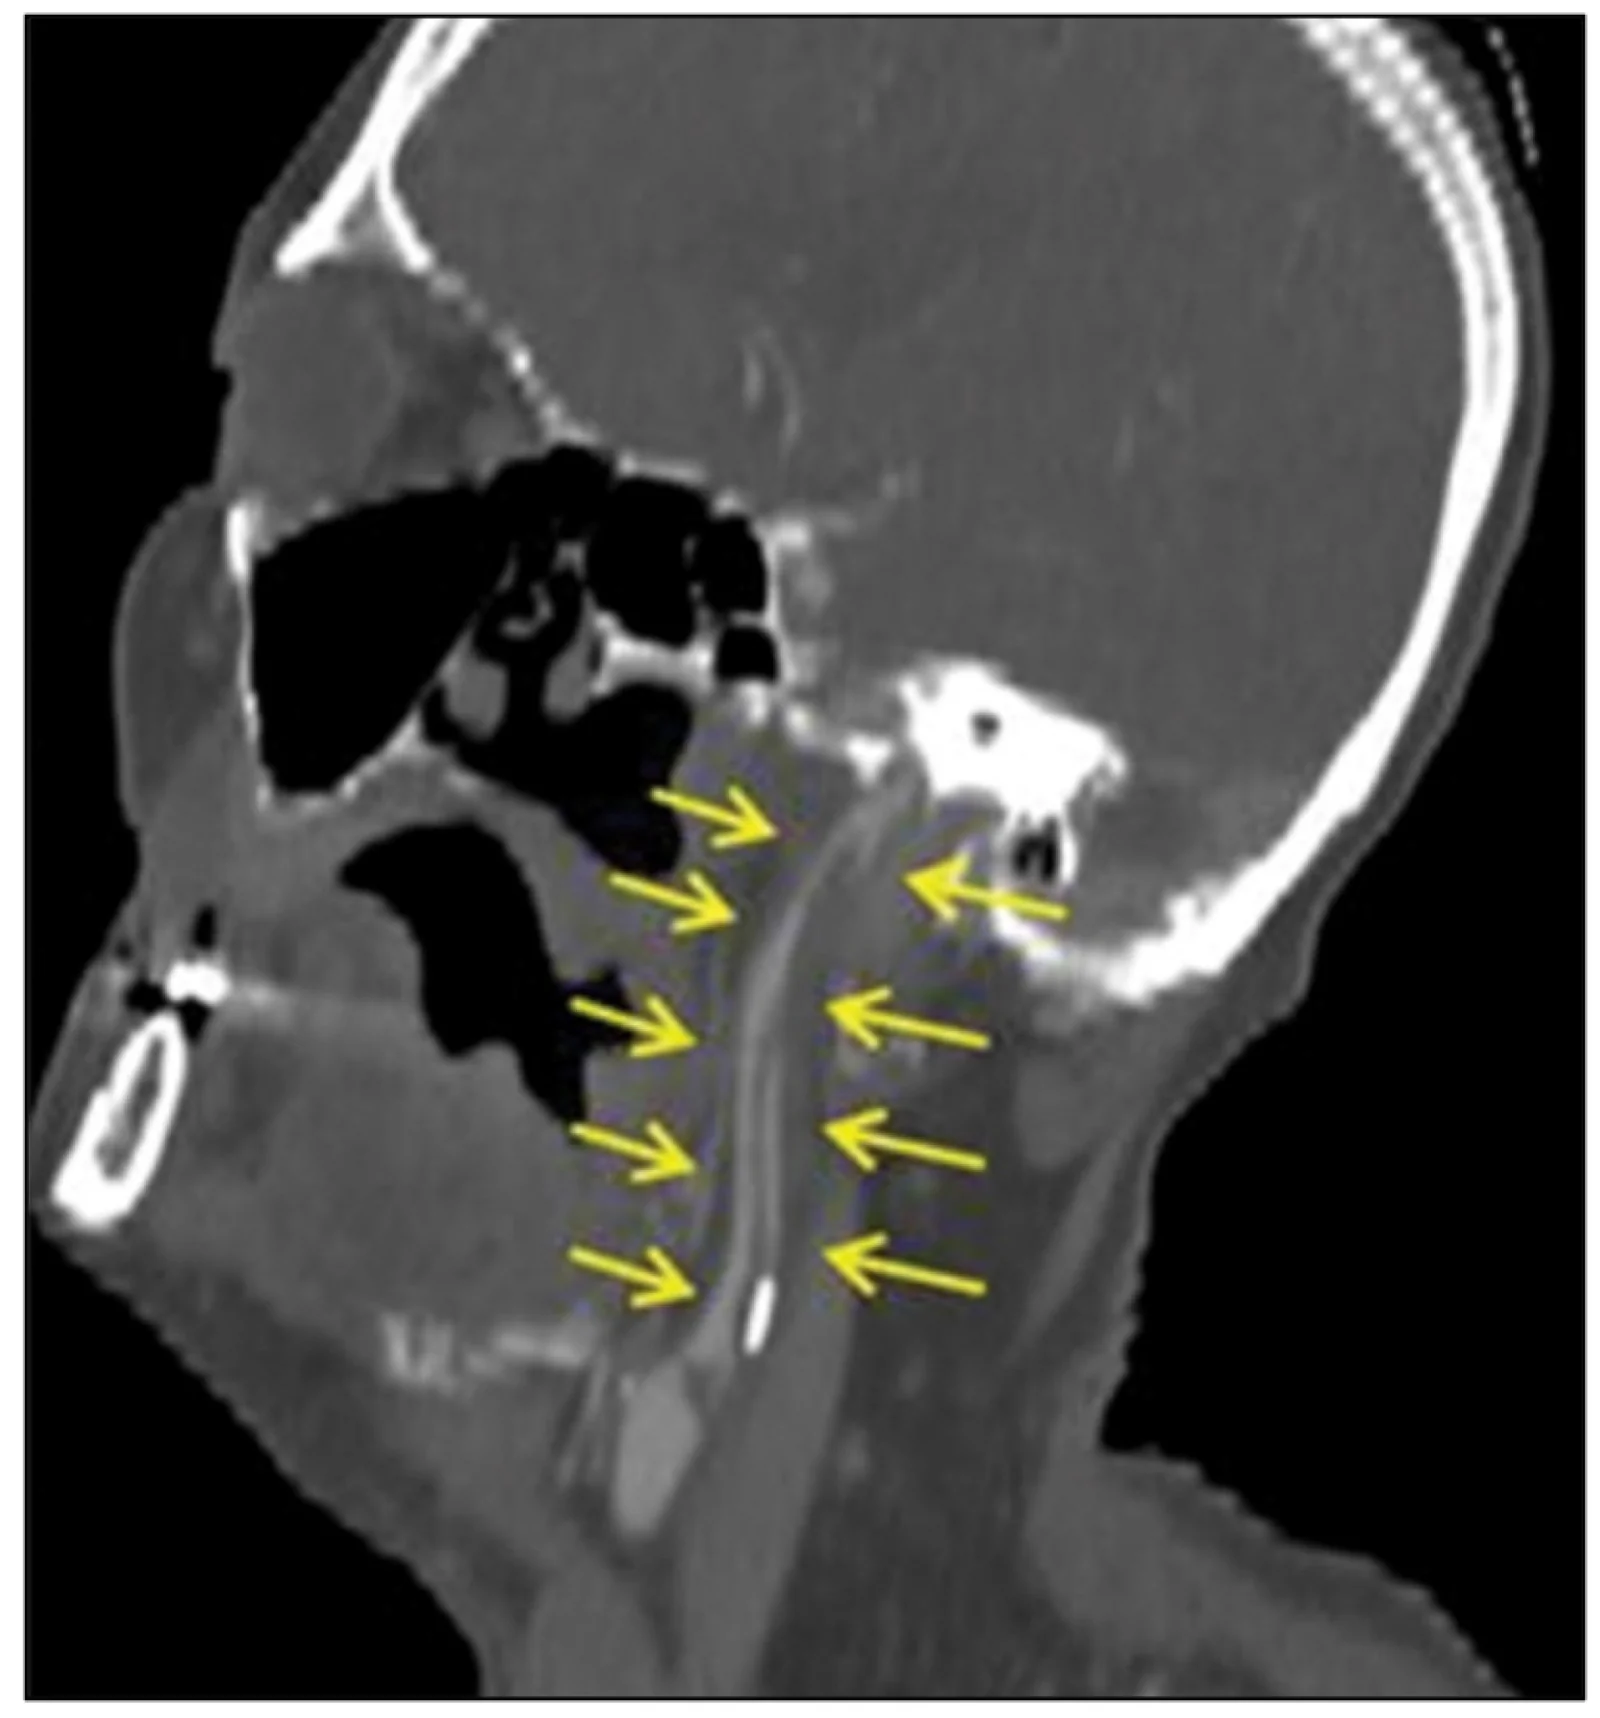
Sagittal computed tomography angiography (CTA) image demonstrating a carotid free-floating thrombus (CFFT) (arrows) attached to the carotid bulb and extending distally to the carotid siphon.
